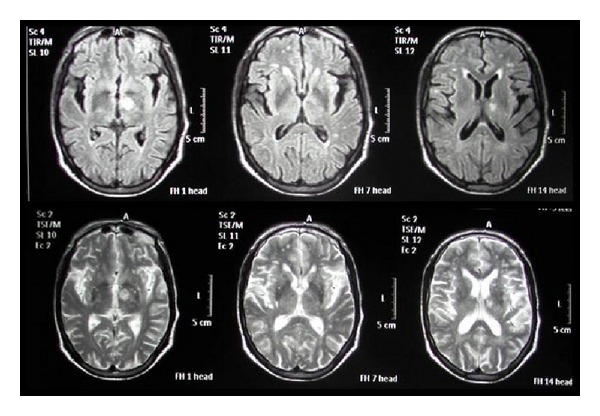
The second MRI (2 days after recent event) revealed another infarct area in left subthalamus.
